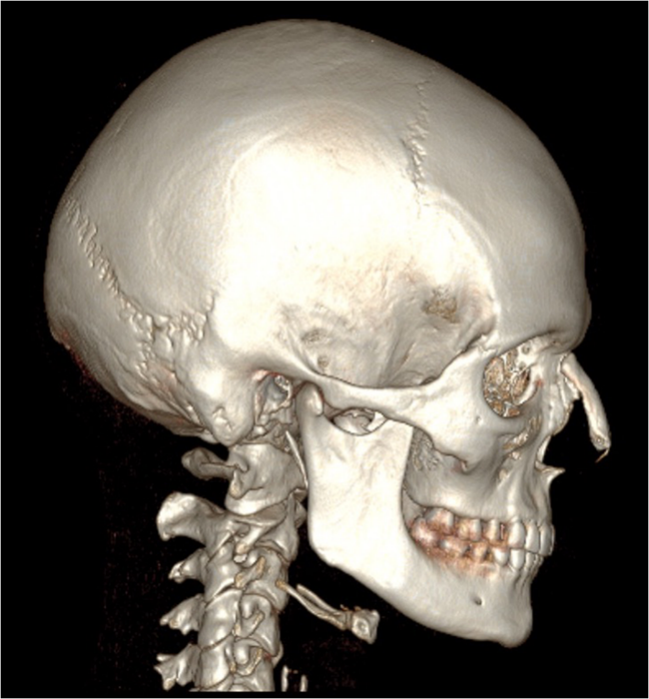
CBCT scan 2 years postoperatively of the patient in Fig. 3
